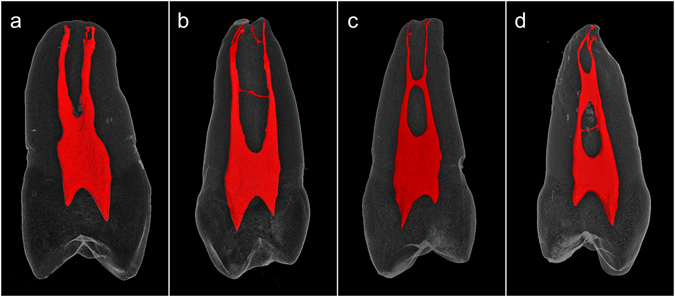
Representative micro-computed tomography reconstructions of single-rooted maxillary premolars containing double root canals. (a) Two separate canals. (b) Two separate canals connected by a lateral anastomosis in the mid-root. (c) Two separate canals connected by a larger anastomosis in the mid-root. (d) Two separate canals connected by a sheet-like isthmus in the middle-third and a narrow anastomosis in the coronal-third of the root.
